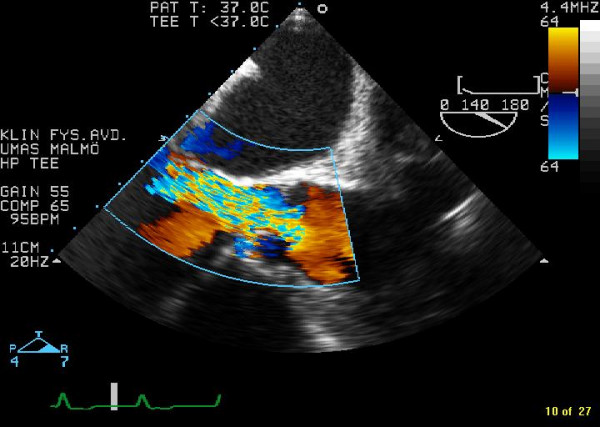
Transoesophageal Colour-Doppler image of severe aortic regurgitation associated with the quadricuspid aortic valve in this case.
